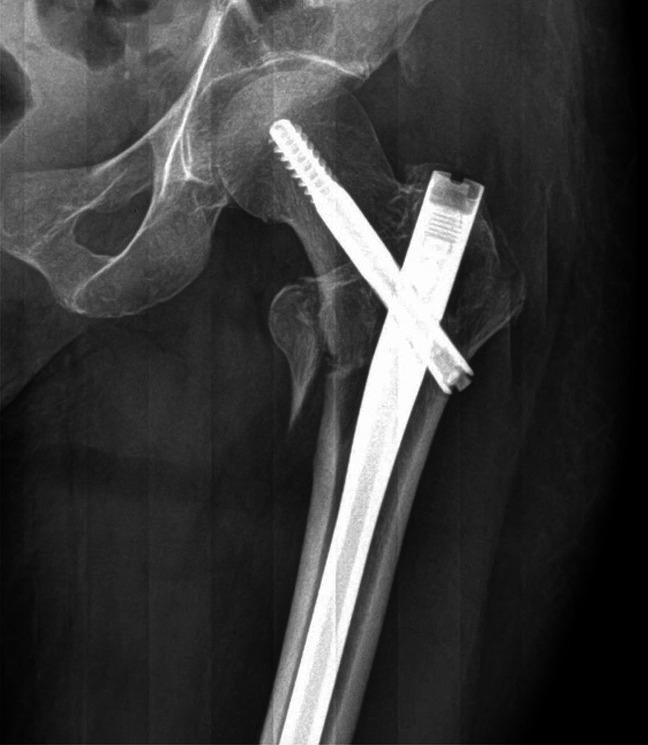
A postoperative AP radiograph of the left hip.
